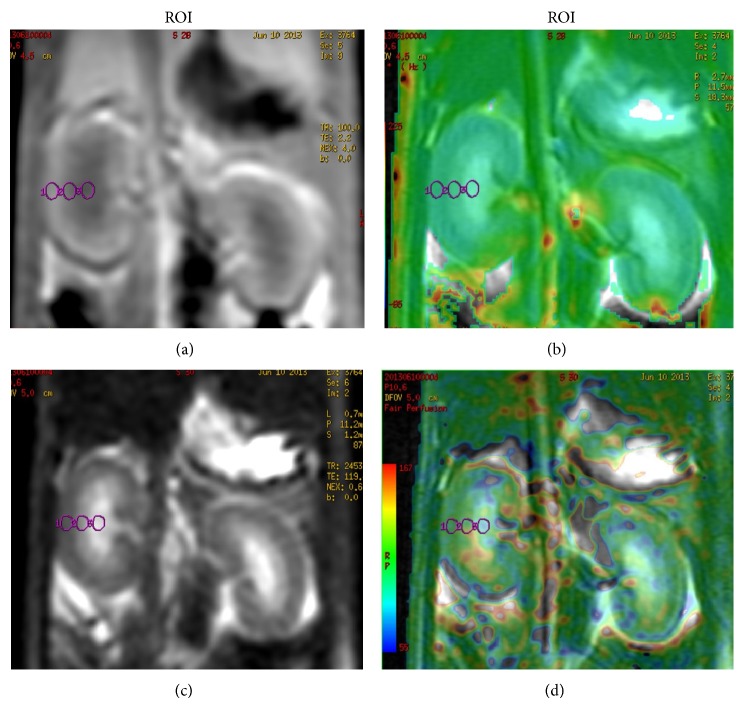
Representative coronal MR image (a) and the *R*
